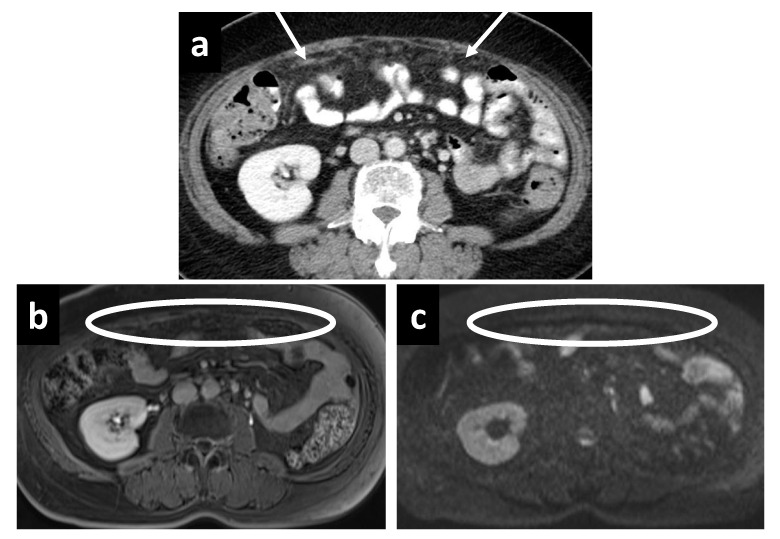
The superior spatial resolution of (a) CT is demonstrated here as irregular soft tissue stranding within the greater omentum, which is not appreciated on (b) delayed and (c) DW-MRI performed on the same day.
